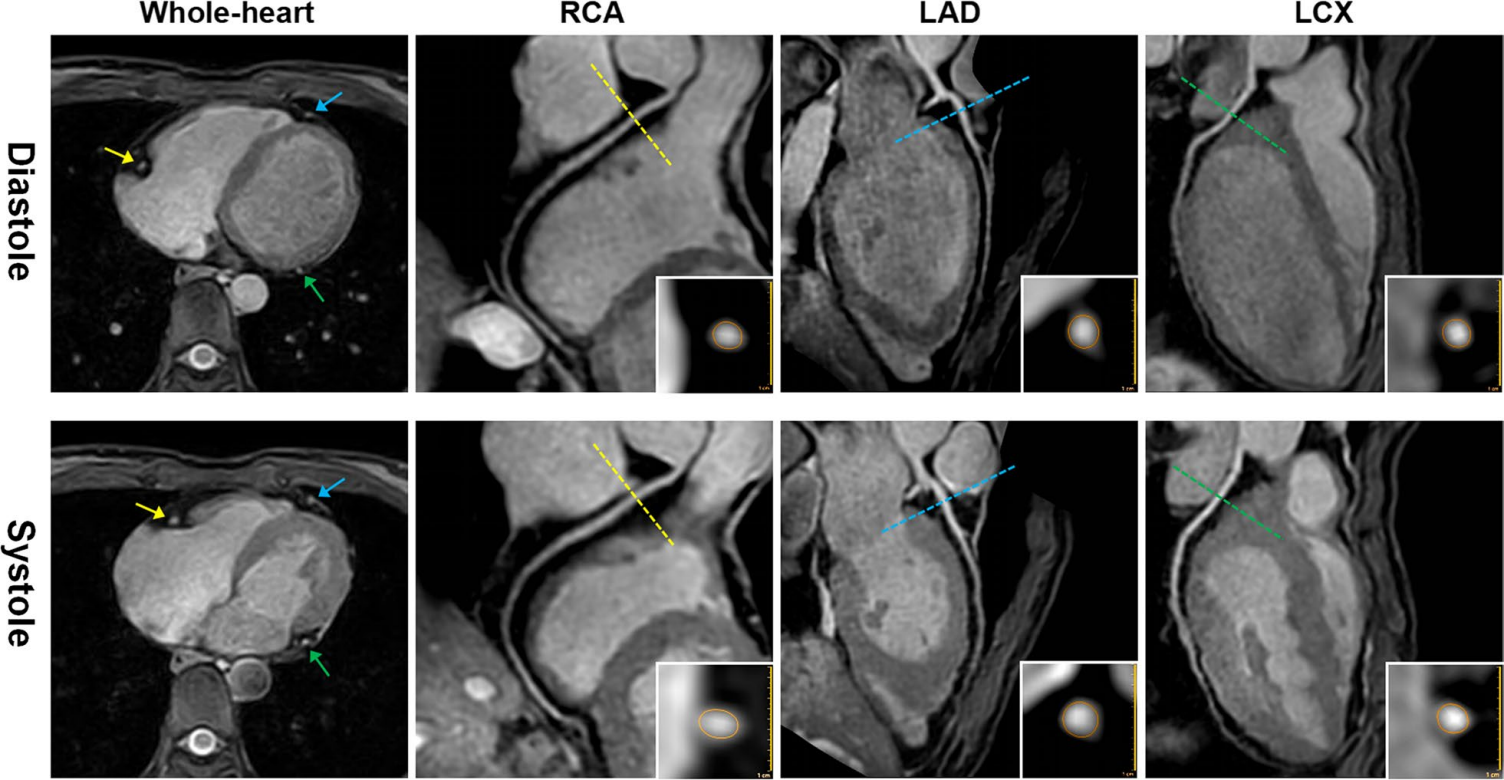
Coronary distensibility of a healthy volunteer measured with coronary MR angiography (MRA). Images in a 26-year-old female healthy volunteer. Peripheral blood pressure was 108/68 mm Hg (pulse pressure = 40 mm Hg). The axial original whole-heart MR images at diastole and systole show the difference in lumen size of right coronary artery (RCA) (yellow arrows), left anterior descending artery (LAD) (blue arrows),and left circumflex coronary artery (LCX) (green arrows). Coronary MRA images show remarkable coronary distensibility in RCA (lumen area 5.89 mm^2^ at diastole and 7.80 mm^2^ at systole, coronary distensibility index (CDI) = [(7.80 − 5.89)/(5.89/40)] × 1000 = 8.11 mm Hg^−1^), LAD (lumen area 7.01 mm^2^ at diastole and 9.73 mm^2^ at systole, CDI = [(9.73 − 7.01)/(7.01/40)] × 1000 = 9.70 mm Hg^−1^) and LCX (lumen area 5.02 mm^2^ at diastole and 6.93 mm^2^ at systole, CDI = [(6.93 − 5.02)/(5.02/40)] × 1000 = 9.51 mm Hg^−1^)
